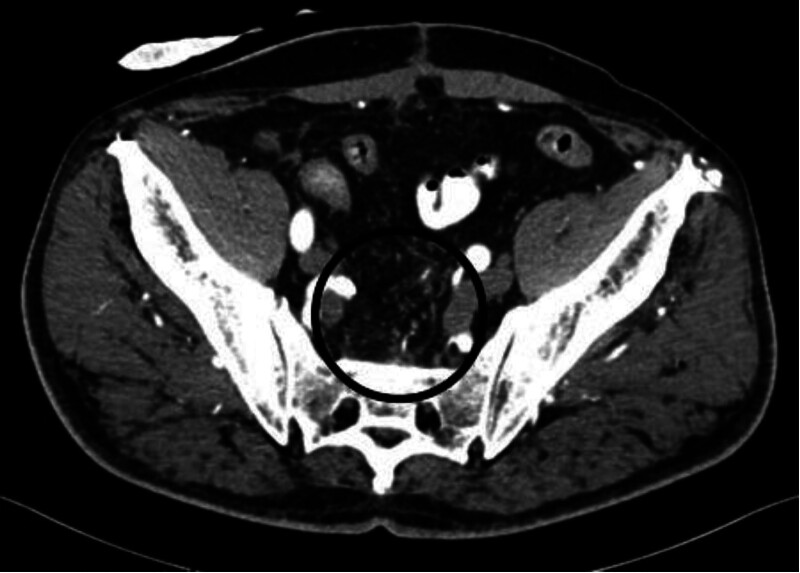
Follow-up CT scan taken 2 months postoperatively showing complete resolution of the hematoma, shown in Figure 5. (black circle). CT = computed tomography.
